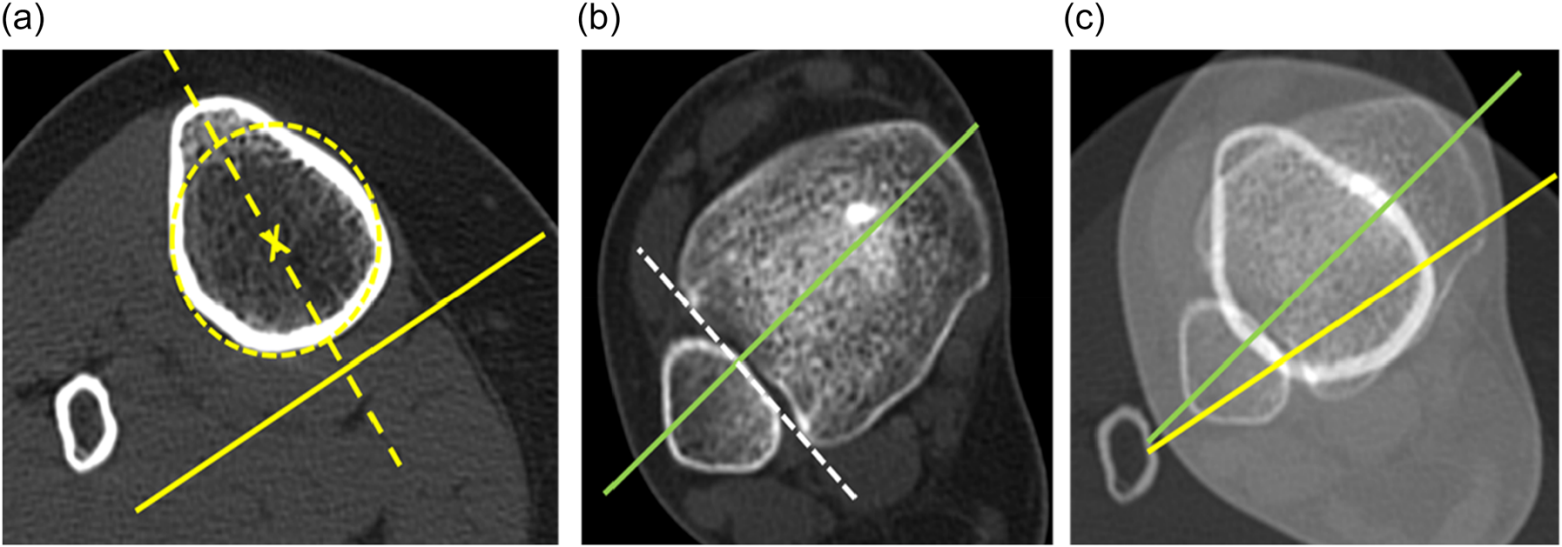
DTT measurement. (a) Axial CT image of the tibia, immediately distal to the TT. The line perpendicular to the line that connects the more anterior point of the tibia (dotted line) with the centre of the circle adapted to the posterior, medial and lateral borders of the tibia represent the proximal line (yellow line). (b) Axial CT image of the tibial plafond and malleoli. The bimalleolar axis is the distal reference line (green line). (c) Addition CT image of both infratuberositary tibia and ankle malleoli. DTT is the angle formed between the two lines. CT, computed tomography; DTT, distal tibial torsion; TT, tibial tubercle.
